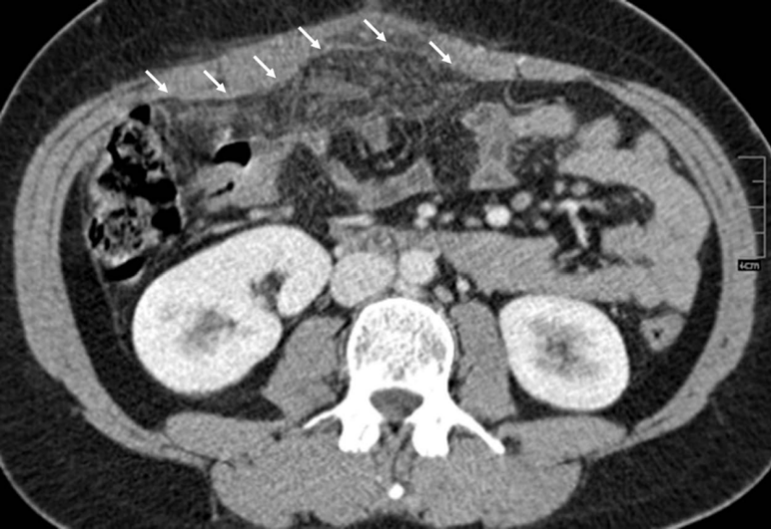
Computed tomography scan of the abdomen showing a hypoperfused mass in the anterior portion of the median epigastrium with fatty density (→) and a thin layer of free fluid surrounding the liver.
